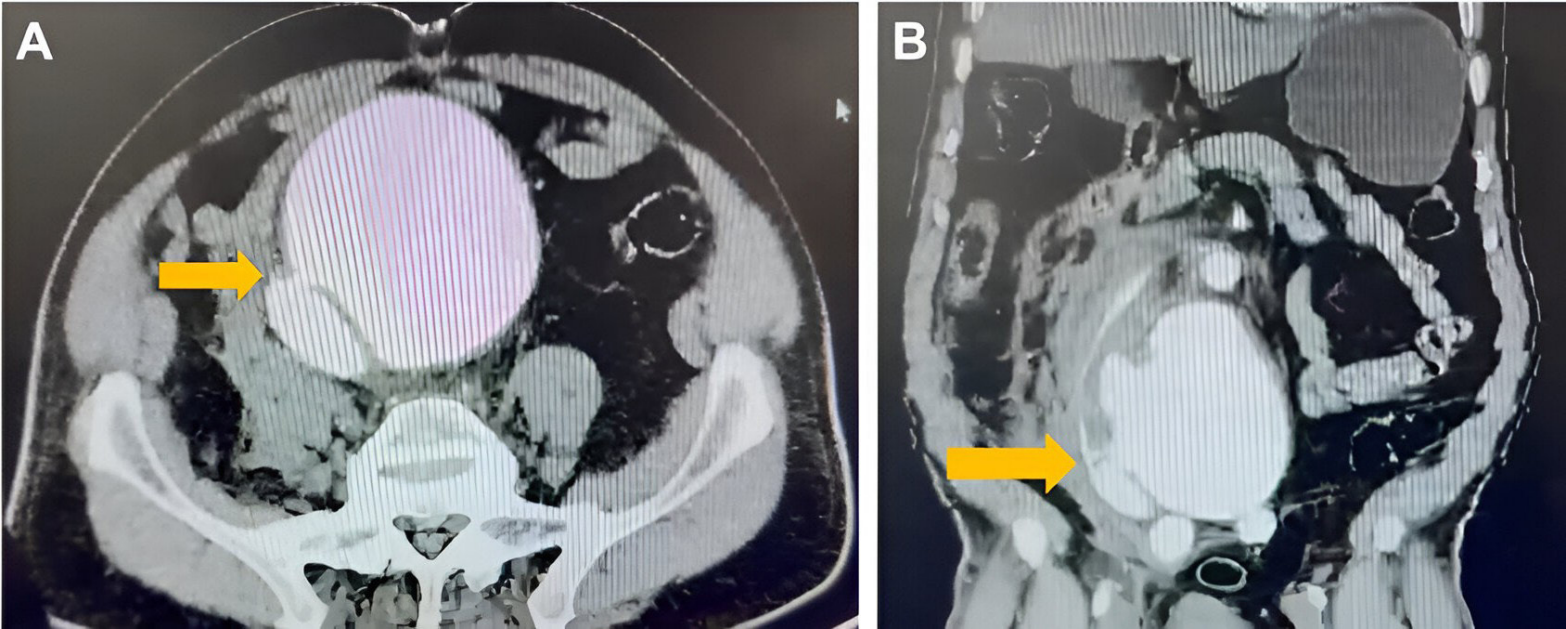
Abdominal computed tomography scan of the patient prior to surgical intervention: (A) axial cut showing the aortocaval arteriovenous fistula (yellow arrow); (B) coronal cut showing the aortocaval arteriovenous fistula (yellow arrow).
